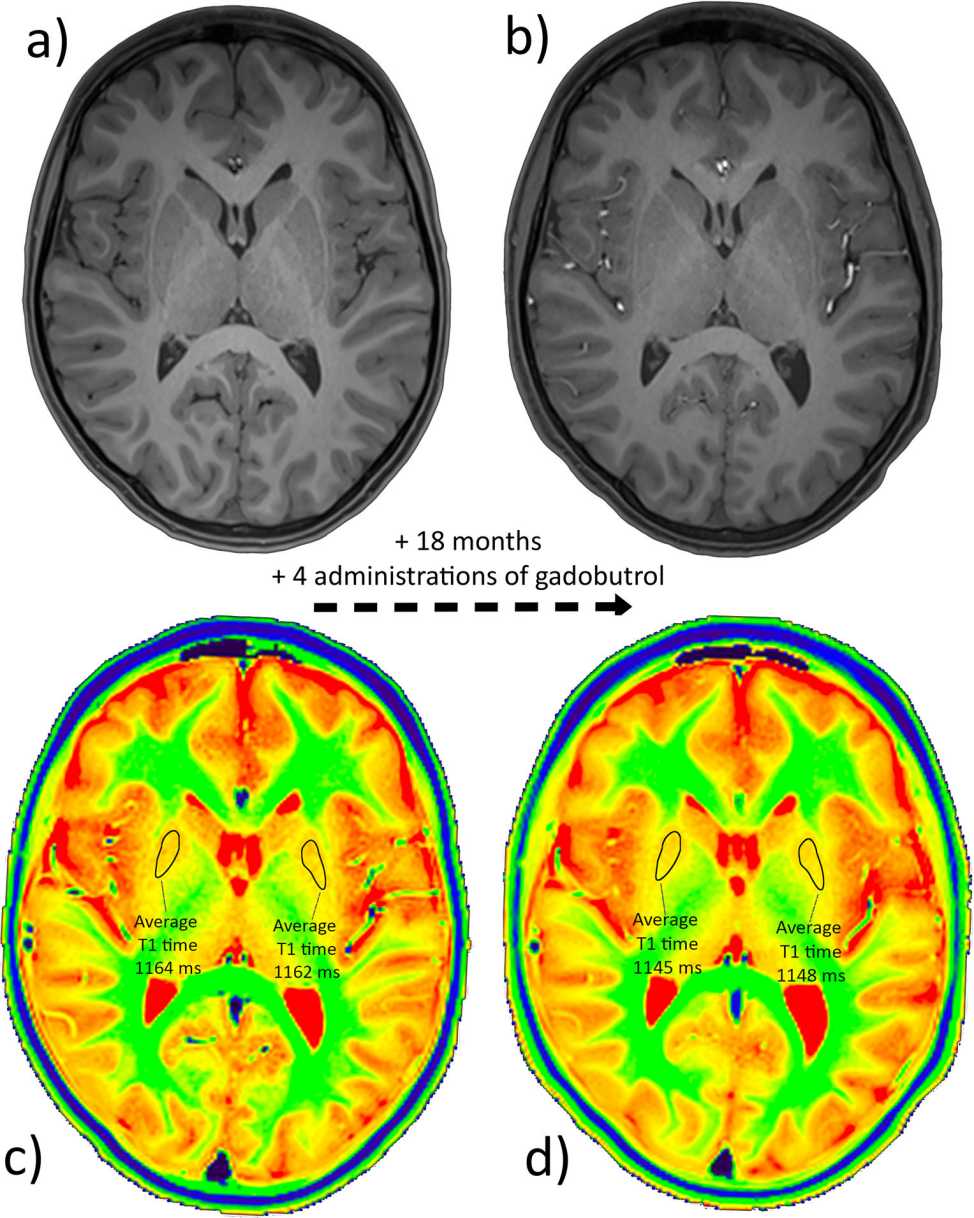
T1-weighted MPRAGE images (a, b) and T1 maps (c, d) at the level of the basal ganglia in a 12-year-old girl with status post cerebellar astrocytoma. An interval of 18 months and four administrations of gadobutrol elapsed between the time point (a, c) and the second time point (b, d). While the T1-weighted images do not indicate a difference (same windowing), a small decrease of the age-adjusted T1 time from − 6.0 to − 13.5 ms can be measured in the T1 maps of the putamen
